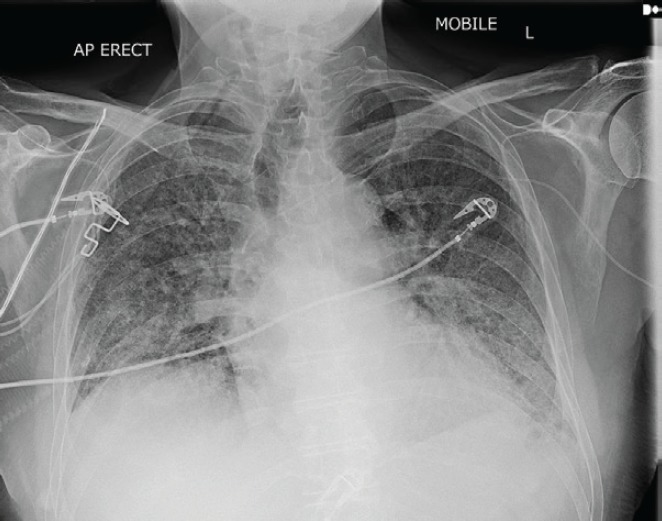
Panitumumab-induced fatal ILD. Chest X-ray on day 17 of admission, showing further deterioration.
